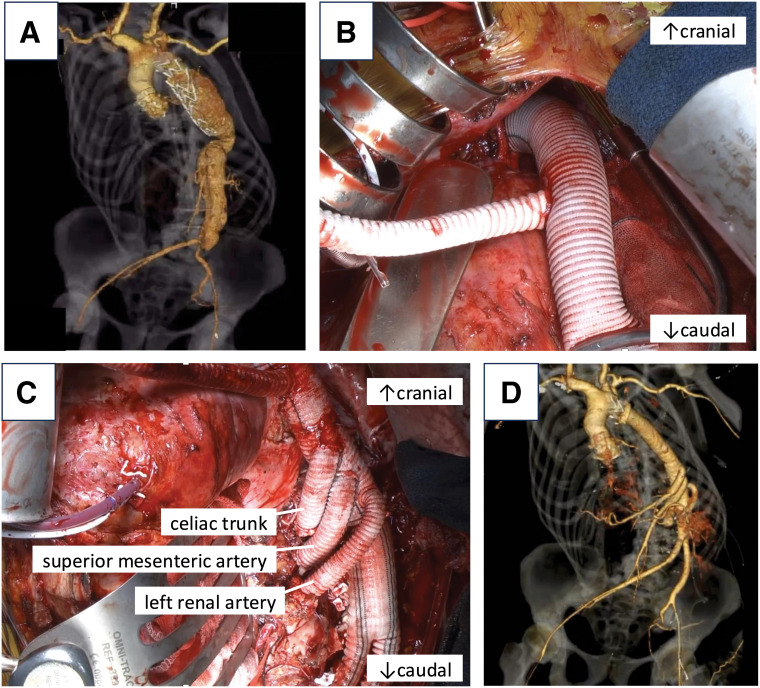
Preoperative imaging, key intraoperative steps, and postoperative outcomes. (A) Preoperative CT angiography showing progressive aneurysmal dilation of the stented aorta. (B) Intraoperative view of the proximal graft anastomosis via SIRC. (C) Reimplantation of the celiac, superior mesenteric, and bilateral renal arteries into the graft. (D) Postoperative CT angiography showing no abnormal findings. SIRC, straight incision with rib-cross thoracotomy
